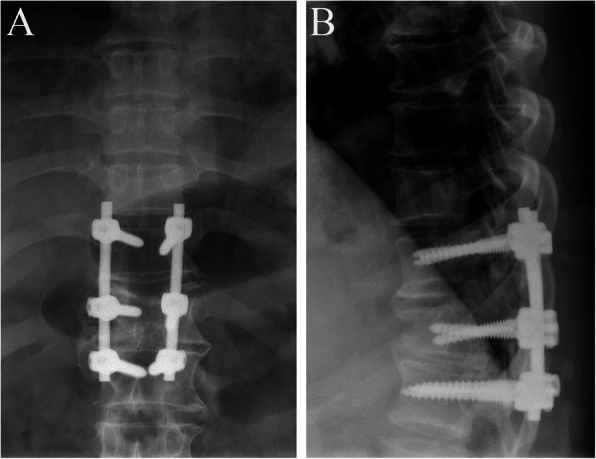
After 12 months of follow-up, radiographic images show no evidence of implant migration or disruption
